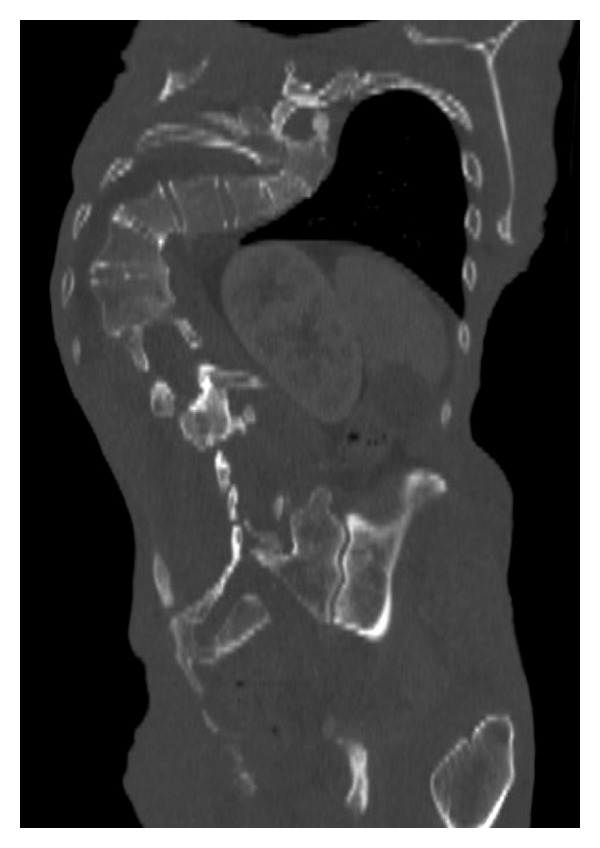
A 53-year-old man with neurofibromatosis type I. A morphostructural abnormality in the spine is characterized by significant dorsolumbar scoliosis with right convexity, as observed in his CT scan (coronal section).
